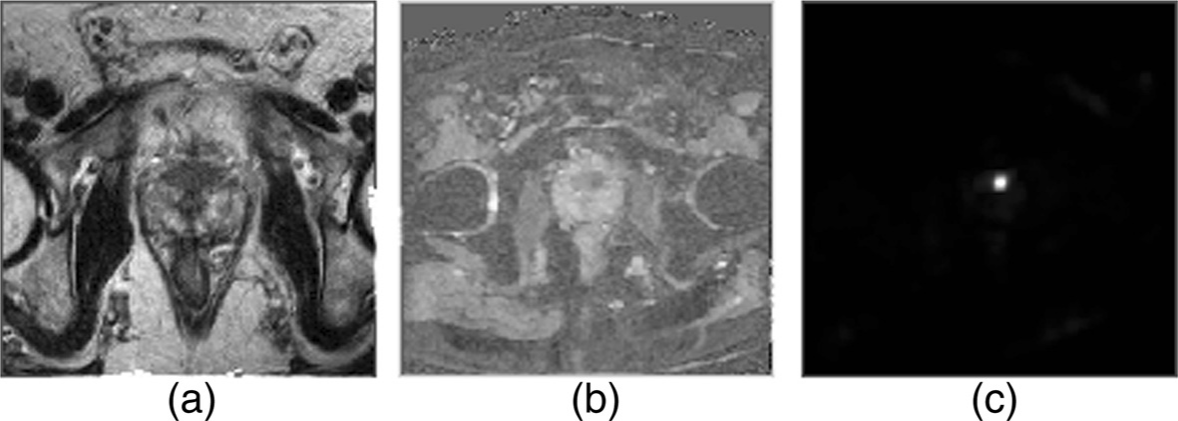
Example case 1. Tumor stands out well on CDI and not at all on T2-weighted imaging in patient with prostate cancer. a), T2-weighted imaging shows no change in signal towards left side of transition zone. b), ADC map shows increased contrast around left side of transition zone. c), CDI shows very high signal intensity corresponding to left side of transition zone.
